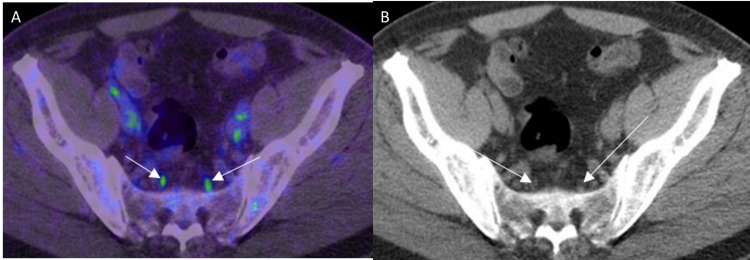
Normal PSMA uptake in the sacral ganglia A: PSMA PET/CT axial image through the pelvis shows radiotracer uptake in the bilateral sacral ganglia (white arrows). B: CT component of PET/CT shows correlate tiny teardrop-shaped soft tissue in the bilateral pre-sacral regions (white arrows) suggestive of the sacral ganglia.
